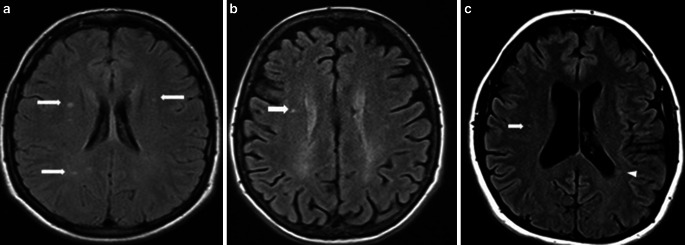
Focal white matter lesions in three PPMS patients. a Axial fluid attenuated inversion recovery (FLAIR) images with only a few small, focal white matter lesions located in deep white matter in right and left brain hemisphere (*white arrows*), b axial FLAIR image with punctate lesion in right deep white matter (*white arrow*), c axial FLAIR image with small, focal lesion located in deep white matter of right brain hemisphere (*white arrow*) and in periventricular white matter (*white arrowhead*) of left brain hemisphere
